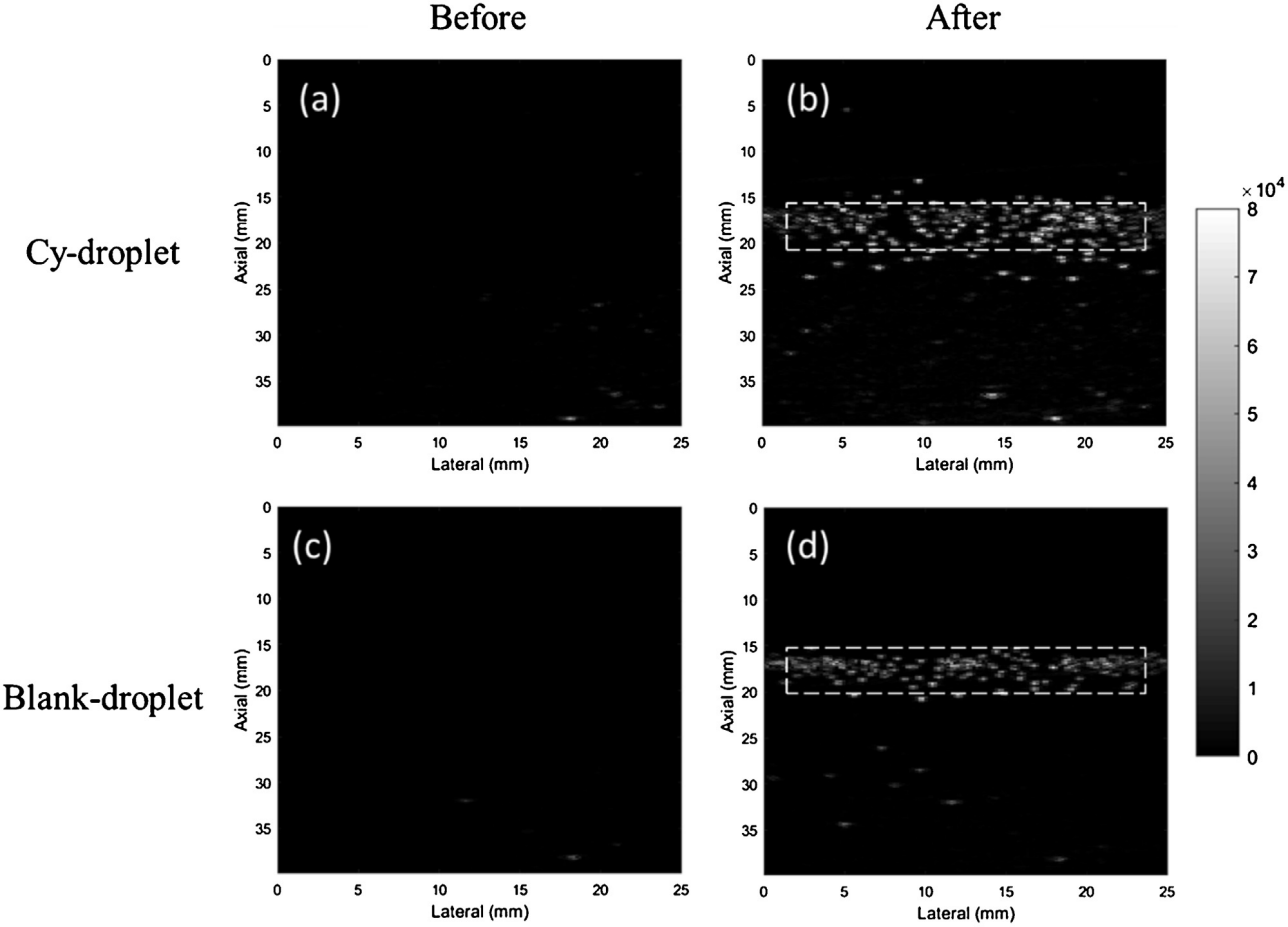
Ultrasound echo enhancement with acoustic vaporisation. (a, b) Cy-droplets and (c, d) blank-droplets (no cyanine dye in the lipid shell). Representative ultrasound images are shown before (a, c) and after (b, d) acoustic vaporisation in a 2L 37 °C water tank. The dashed white rectangular area was the ROI applied to quantify the echo strength.
